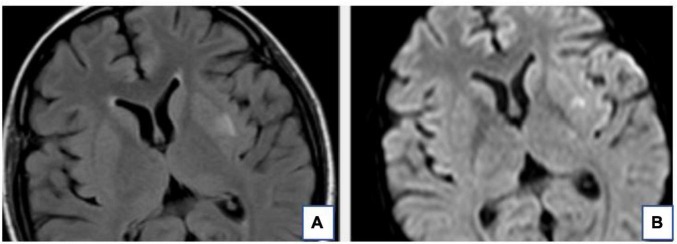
The T2-FLAIR (A) and DWI (B) of the same patient. The hyperintense signal of the Left basal ganglia area on T2-FLAIR is also very obvious on DWI. This suggests local infarction in the left basal ganglia.
